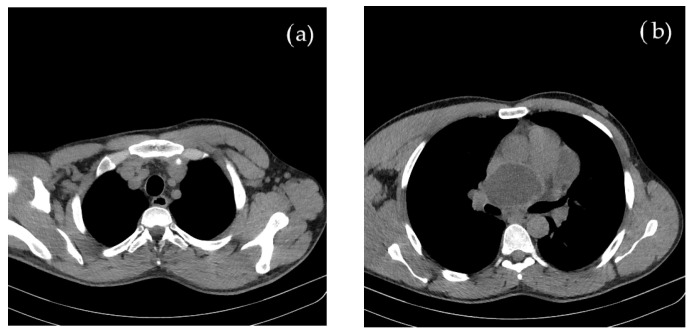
Contrast and native CT of thorax and abdomen shows multiple (a) mediastinal and (b) pericardial hydatid cysts.
